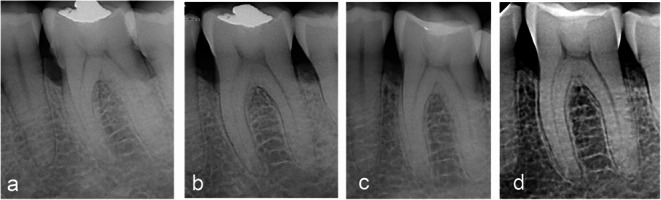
Treatment of an intrabony defect at a lower jaw molar with EMD. a preoperative radiograph. b 6 months post-surgically partial fill of the intrabony component. c at 1 year defect fill is clearly visible. d at 5 years complete defect fill and stability
